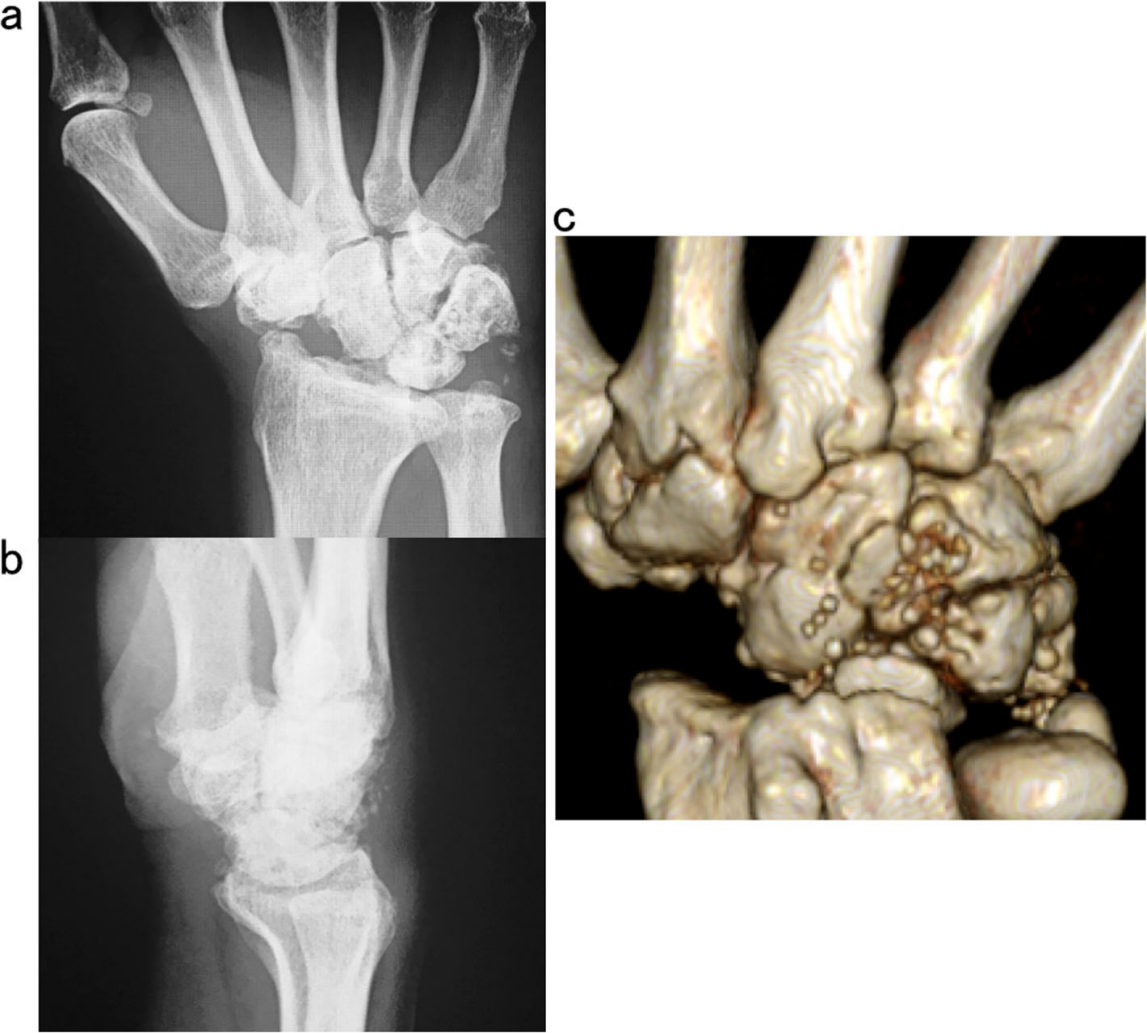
X-rays (a, b) and three-dimensional computed tomography scan. Fifty-six-year-old male patient 5 years after the operation. Note that the four corners have united and the BAP has almost disappeared. BAP, bioabsorbable plate
